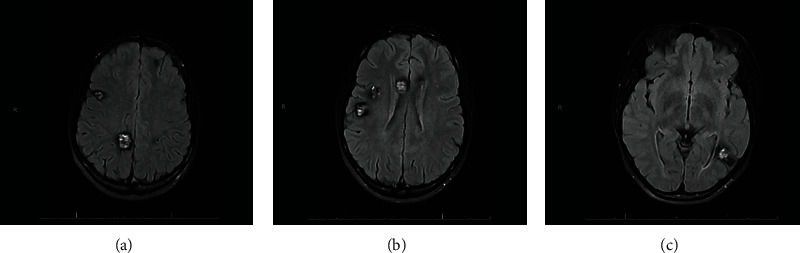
Axial T2 FLAIR sections (superior to inferior) from an MRI performed upon the patient's arrival to the hospital. Various cavernomas are present. The largest lesion in the right cerebral hemisphere is in the paramedian right parietal lobe and measures 15 × 18 mm on axial images and 17 mm superior to inferior (a). The largest lesion is in the posterior left temporal lobe near the temporal occipital junction and measures approximately 19 × 16 mm on axial images and 17 mm superior to inferior (c).
